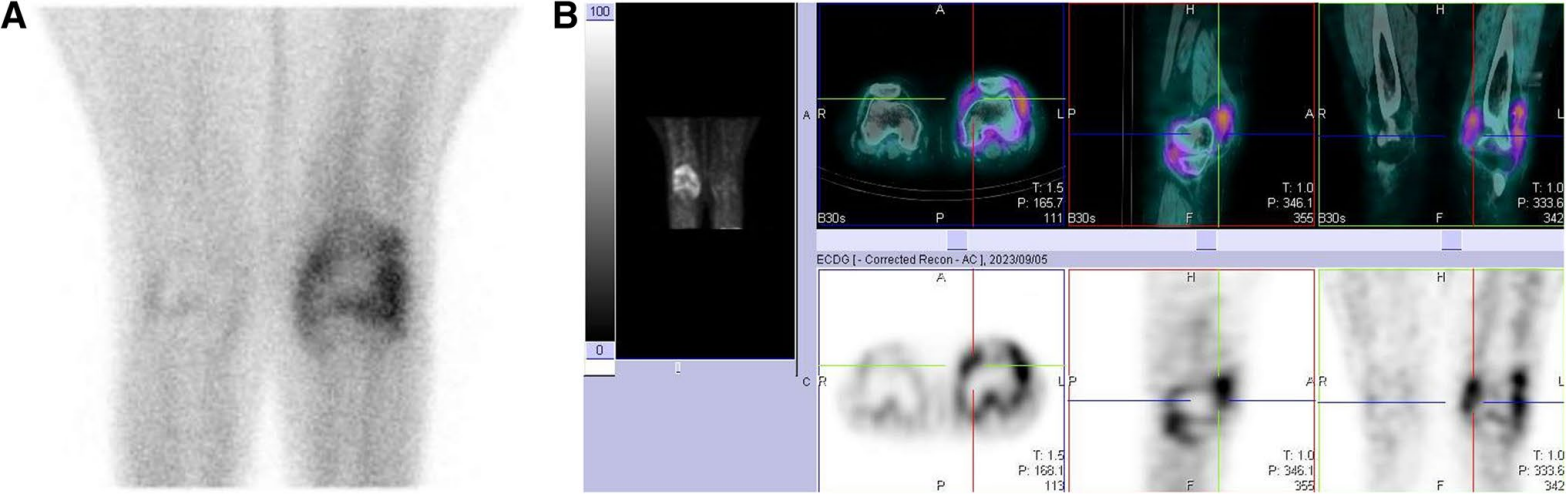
Delayed static (A) and SPECT/CT images of a 51 year old with unilateral knee disease. Note the normal physiologic uptake of radiotracer in the right knee
